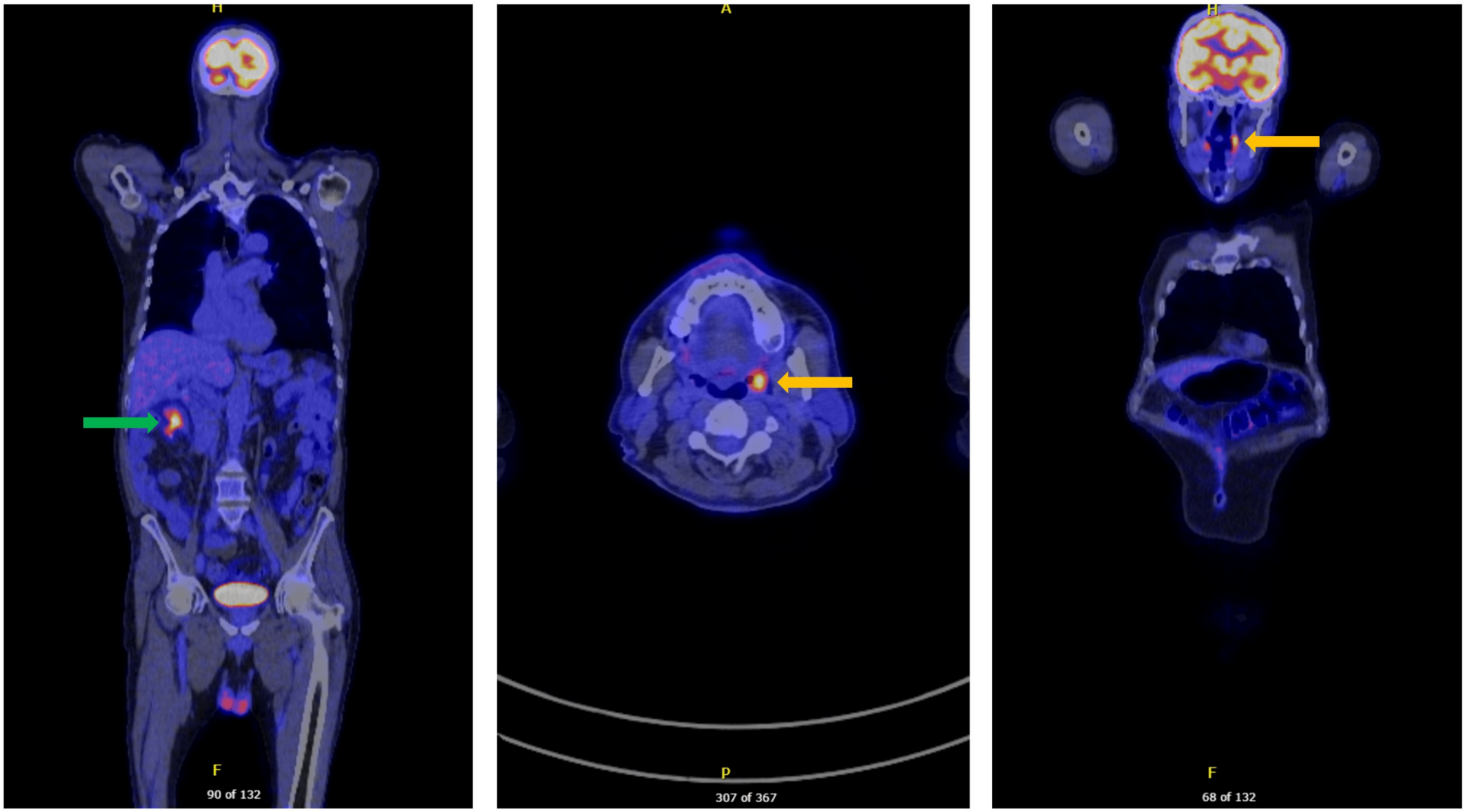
Post-operative initial PET-CT The yellow arrow indicates fluorodeoxyglucose (FDG) uptake in the left tonsil, while the green arrow indicates physiological FDG uptake in the colon. PET-CT: Positron emission tomography-computed tomography.
